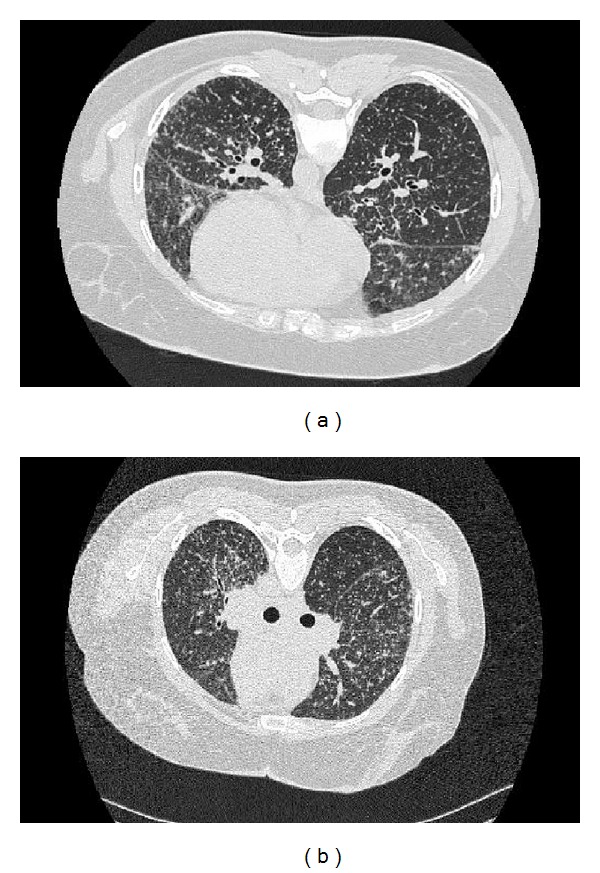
High resolution computed tomography showed multiple small nodules throughout the lung, few peribronchovascular nodules, with multiple enlarged mediastinal and hilar lymph nodes.
